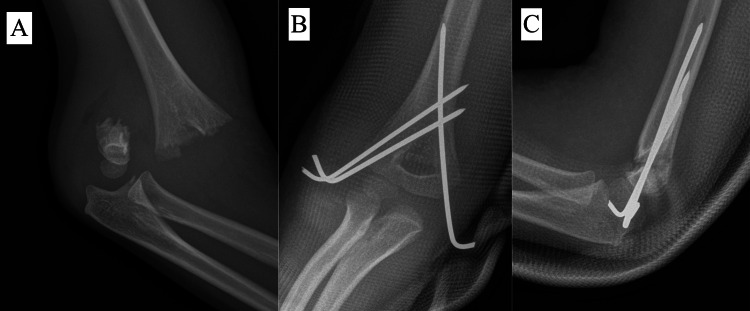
Radiograph of a four-year-old patient with Type IV supracondylar fracture (A) Preoperative radiograph. (B) Postoperative AP view showing other pin configurations. (C) Postoperative lateral view.
